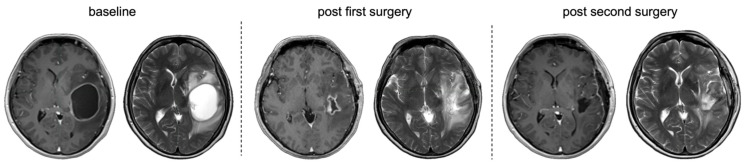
MRI from a representative case with primary debulking and supramaximal resection after two-stage surgery. From left to right. T1-CE and T2-nCE baseline to post second surgery. Postoperative MRI after the first surgery on a cystic left parieto-temporal lesion demonstrating residual T1-CE as well as T2-nCE residual tumour. MRI after the second surgery shows no residual tumour.
